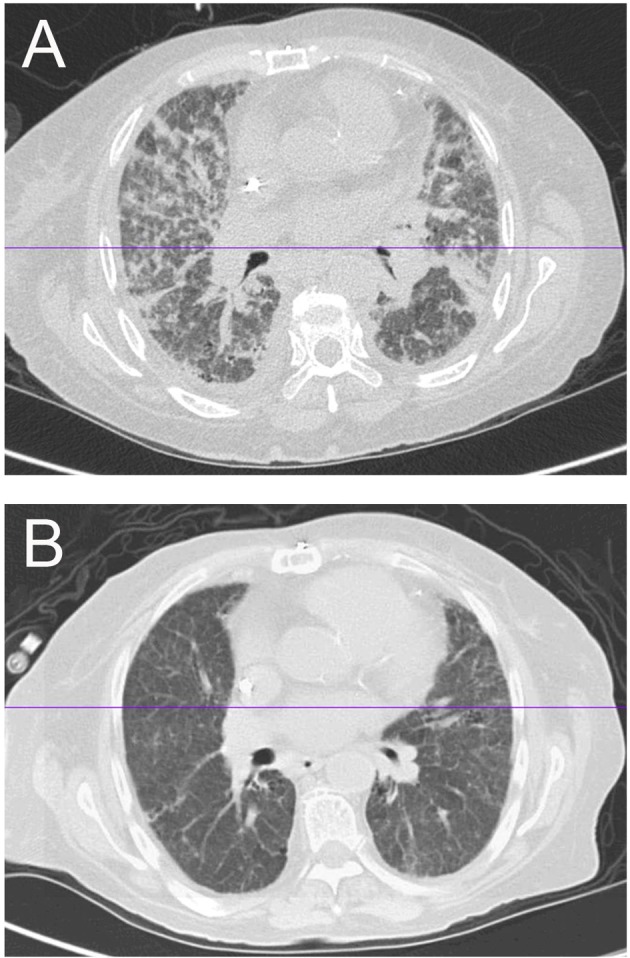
Comparison of imaging before and after treatment (plasmaphoresis). (A) HRCT showing alveolar and interstitial pulmonary edema of bilateral lung fields. (B) CT of thorax showing interval resolution of the alveolar and interstitial pulmonary edema after plasmaphoresis.
